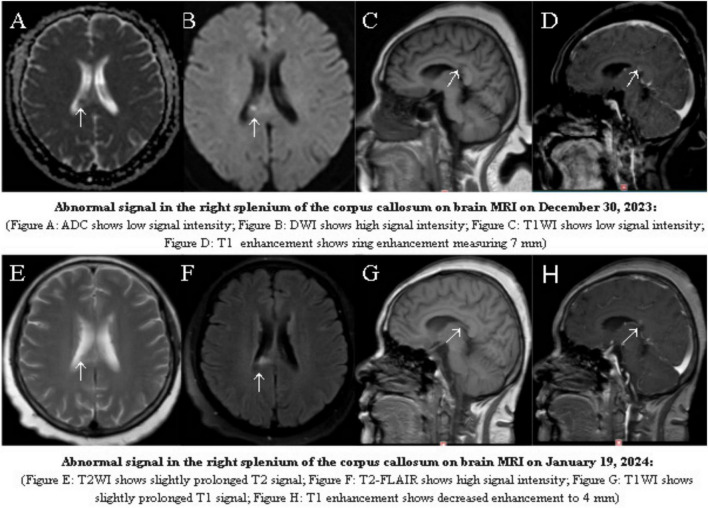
Abnormal signal in the right splenium of the corpus callosum on brain MRI on 30 December 2023: (A) ADC shows low signal intensity; (B) diffusion-weighted imaging (DWI) shows high signal intensity; (C) TIWI shows low signal intensity; (D) T1 enhancement shows ring enhancement measuring 7 mm). Abnormal signal in the right splenium of the corpus callosum on brain MRI on 19 January 2024: (E) T2WI shows slightly prolonged T2 signal; (F) T2-FLAIR shows high signal intensity; (G) TIWI shows slightly prolonged T1 signal; (H) Tl enhancement shows decreased enhancement to 4 mm).
